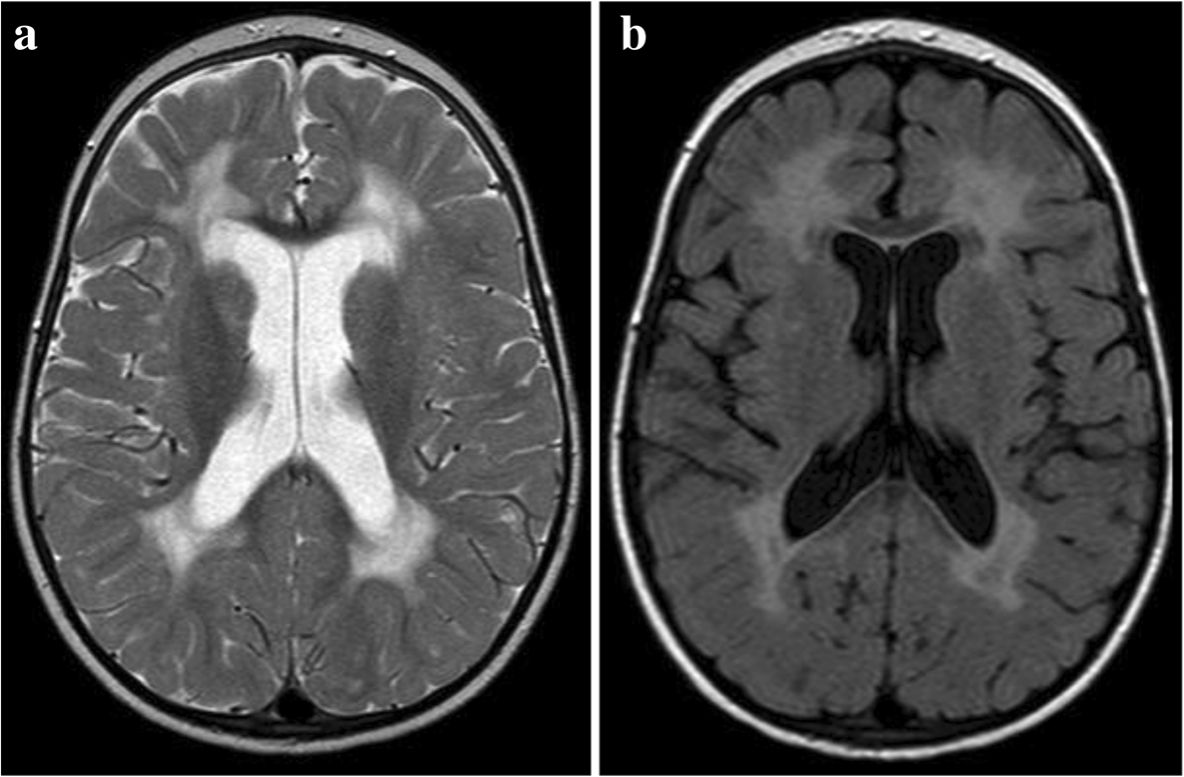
Axial T2 (a) and FLAIR (b) images of an HIV-positive child showing bilateral symmetrical high signal in the periventricular white matter with normal basal ganglia. Prominence of the cerebral sulci implies volume loss.
